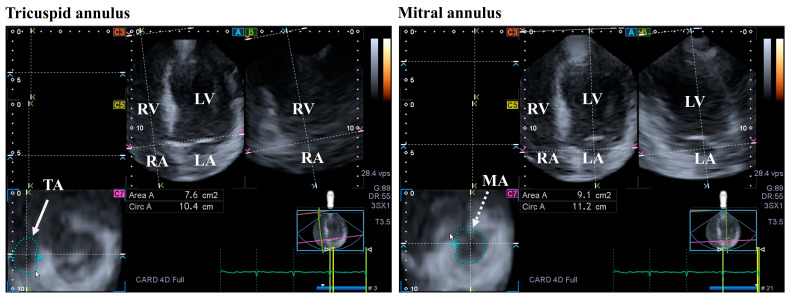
Evaluation of the tricuspid (TA) and the mitral annuli (MA) by three-dimensional (3D) speckle-tracking echocardiography. From the acquired 3D echocardiographic dataset, the following views are created: apical four-chamber (A) and two-chamber views (B) and a cross-sectional view at the level of the TA/MA optimized in apical four- and two-chamber views (C7). The white arrow represents the two-dimensionally projected TA plane, while dashed white arrow represents the two-dimensionally projected MA plane. Abbreviations: RV = right ventricle; RA = right atrium; LV = left ventricle; LA = left atrium; Circ = TA/MA perimeter; and Area = TA/MA area.
